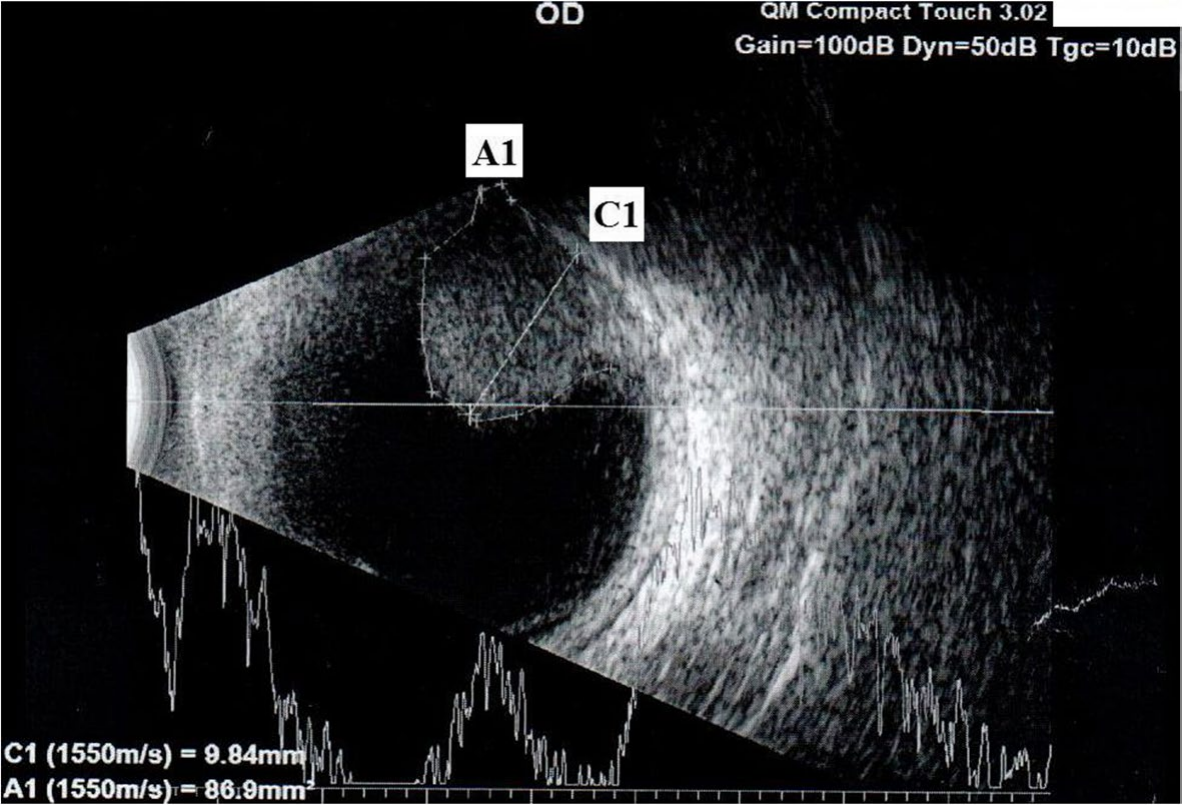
Examples of ultrasound findings in patient with uveal – choroidal melanoma. Left: large melanoma, C1 – elevation of the tumor (9.84 mm), A1 - area of the tumor (86.9 mm^2^), volume of the tumor was 0.8 cm^3^ (Source: Ultrasound Quantel Medical Compact Touch located at the workplace of the authors)
